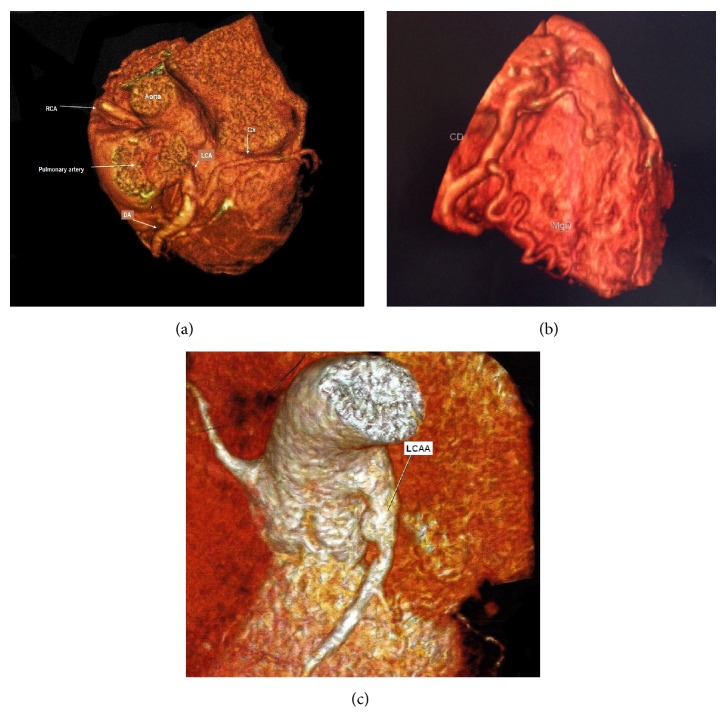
(a) Computed tomography (CT) angiography showing the left coronary artery (LC) from the pulmonary trunk (RCA: right coronary artery; AD: anterior descending artery; and Cx: circumflex artery). (b) CT angiotomography showing the coronary collateral network. (c) CT angiotomography showing the left coronary artery aneurysm.
